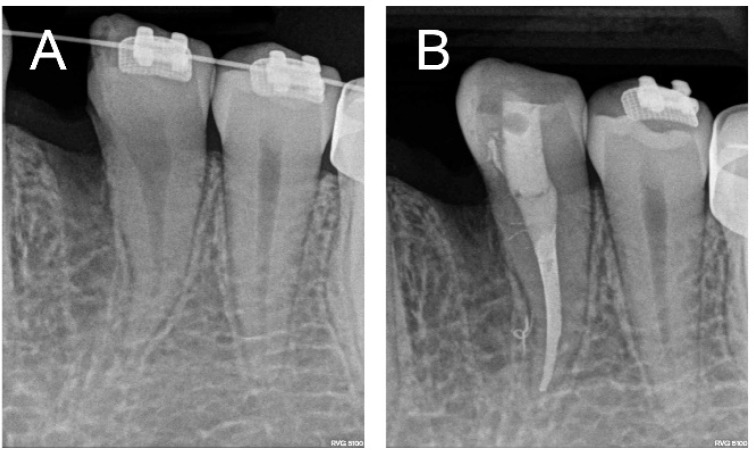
Hemisection and extraction of the mesial portion of the premolar; *A)* Periapical radiograph before endodontic treatment; *B)* Periapical radiograph after endodontic treatment*
